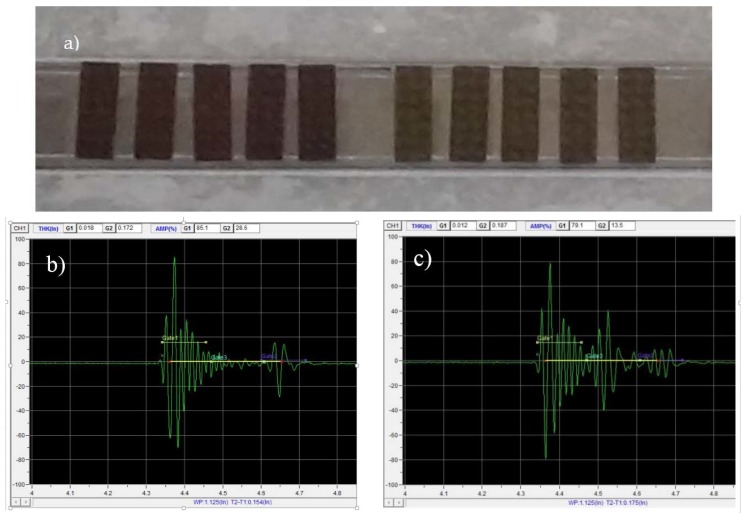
(a) Image of short-beam samples aligned in immersion tank for ultrasonic testing; (b) ‘A-scan’ data from undamaged area of a sample. (c) ‘A-scan’ data from damaged area of a sample.
